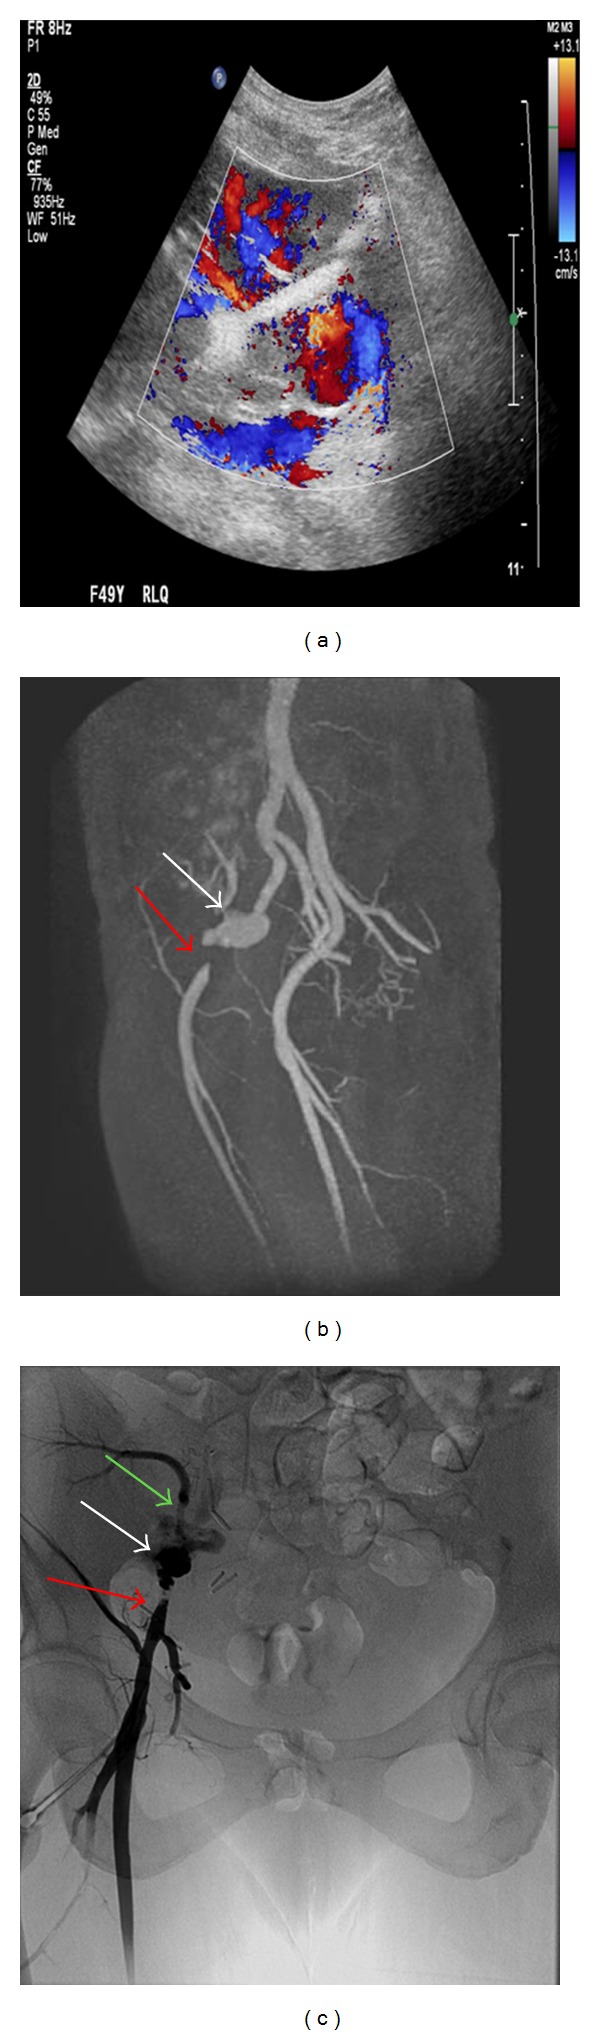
A possible pseudoaneurysm in the region of the vascular anastomosis revealed by Color Doppler Ultrasound (a) with an area of turbulent and to-and-fro waveforms. Follow-up MRI (b) confirmed a bilobed pseudoaneurysm (white arrow) and revealed a segment of high-grade stenosis of the right external iliac artery (red arrow). Conventional angiogram image (c) in AP view reconfirmed the pseudoaneurysm (white arrow) and a segment of high-grade stenosis of the right external iliac artery (red arrow) with stenosis of the transplanted renal artery (green arrow).
